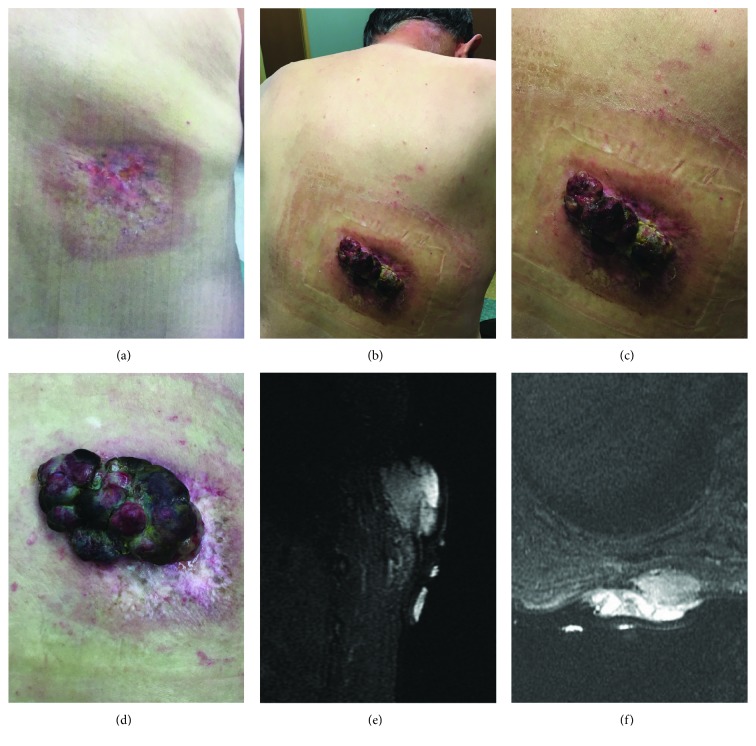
Gross imaging of the patient's mass: gross images of the area of ulceration which developed following cardiac catherization photographed in 2014 (a) show no signs of growth. In 2017, a mass (b) demonstrating outward growth and the area of the radiation injury following a year of close observation with a superimposed image of the same (c) mass was obviously exophytic and purulent measuring 5 × 3 cm at the time. The mass was photographed again prior to surgery two months later (d) in addition to MRI imaging shown with sagittal (e) and axial STIR (f) imaging demonstrating T1 signaling slightly intense to muscle and heterogeneous T2 signaling with avid contrast enhancement at the level of the posterior T10 rib. Both images fail to show evidence of muscular or bony invasion.
